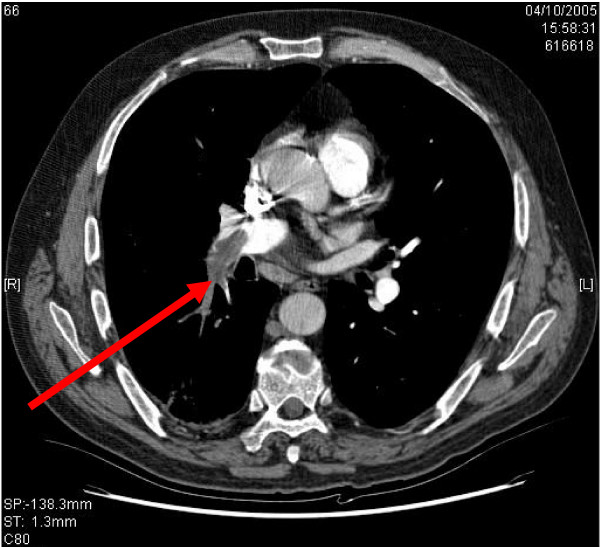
A Computed Tomography Pulmonary Angiogram showing the filling defect associated with a pulmonary embolism (red arrow).
